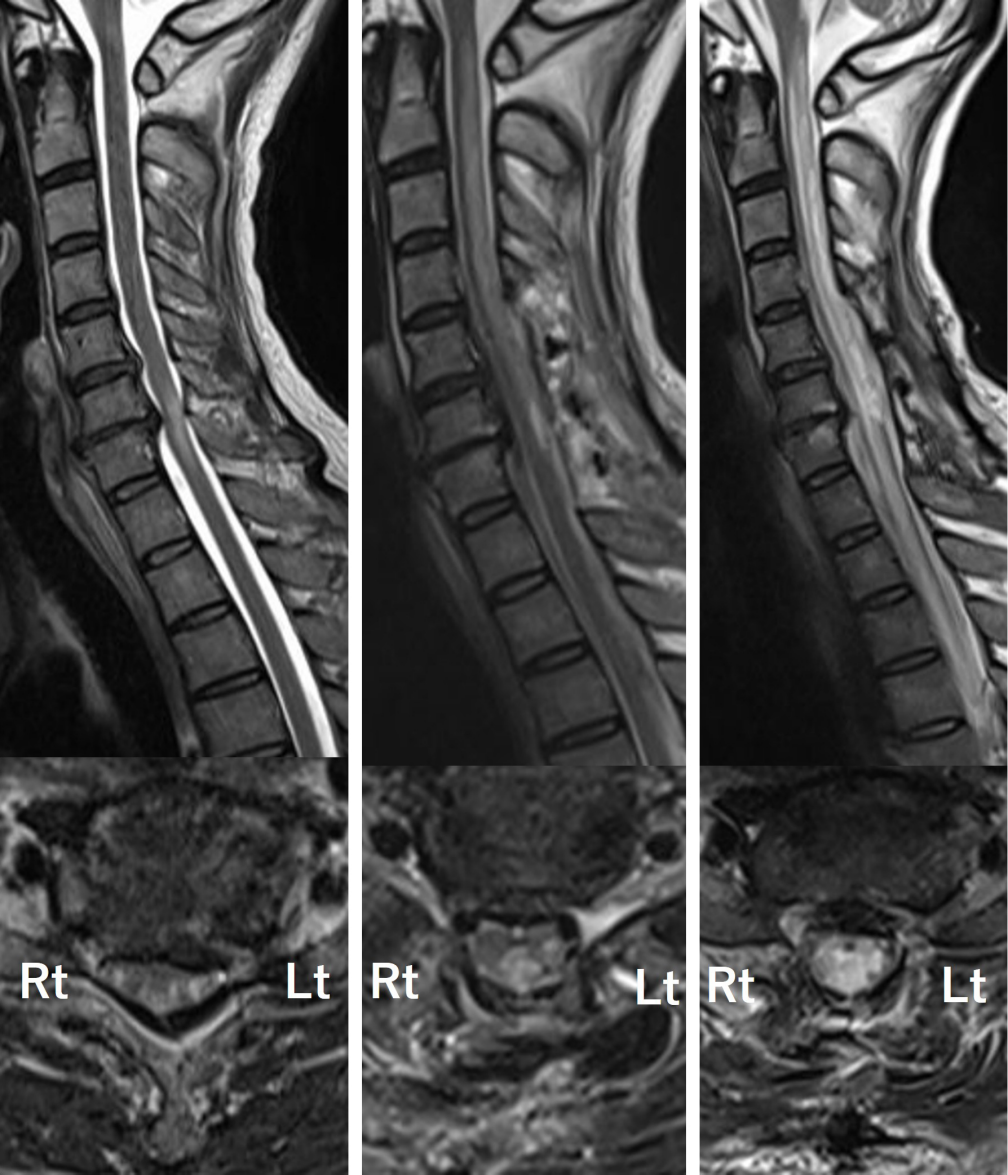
Upper row: Sagittal T2-weighted images of cervical spine MRI over time. Lower row: Axial T2-weighted images at the C6/7 level. A. Preoperative cervical MRI. Signal changes due to spinal cord compression are observed at the C6 and C7 levels, findings consistent with cervical spondylotic myelopathy. B. Cervical MRI 15 days postoperatively. The images show sustained decompression of the spinal cord; however, new T2-weighted hyperintensity lesions are observed in the dorsal columns, extending from the lower medulla to the C7 level. C. Cervical MRI 45 days postoperatively. Despite treatment with intravenous methylprednisolone, the lesions were resistant to therapy. The patient’s symptoms progressively worsened after each dialysis session, which correlated with the expansion of abnormal signal intensity on MRI. MRI: magnetic resonance imaging.
